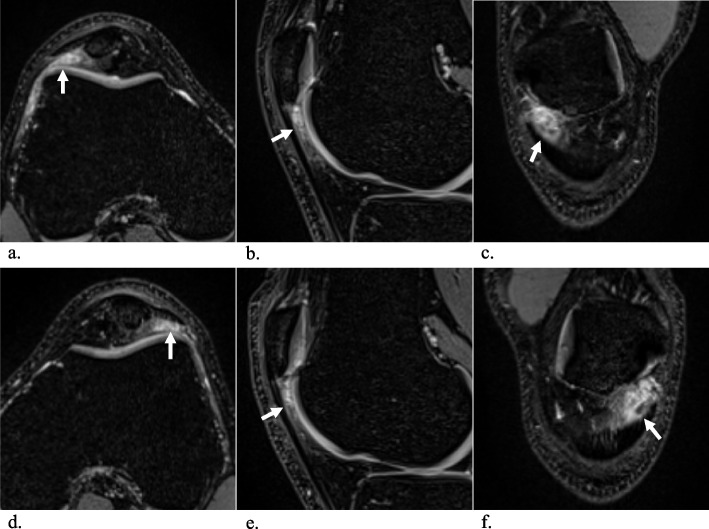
Multiplanar MRI of bilateral superolateral Hoffa fat pad (SHFP) edemas (arrows) of an 18-year-old youth competitive alpine skier (a–c axial, sagittal, and coronal images of the right knee; d–f axial, sagittal, and coronal images of the left knee). SHFP edema was defined as focal high signal intensity in the superolateral corner of the Hoffa fat pad on the fat-suppressed T2-weighted images
